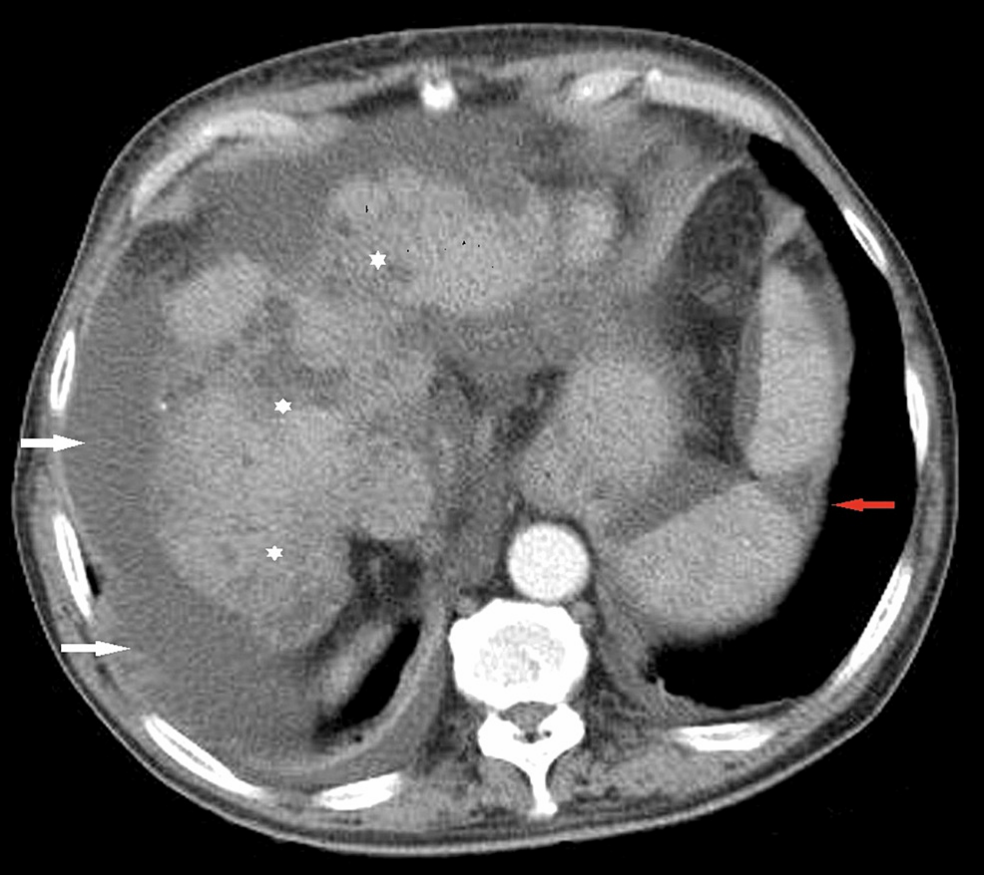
Abdominal CT scan showing a highly cirrhotic liver with surface nodularity and parenchymal heterogeneity (white asterisks) and ascitic fluid (white arrows). The triangular hypodense area indicates the splenic infarction (red arrow).
